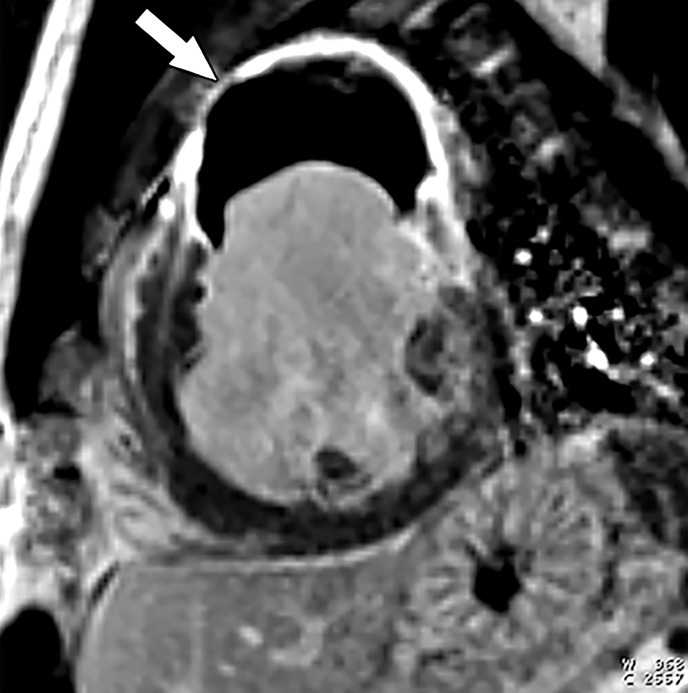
True aneurysm in a 42-year-old man with cardiomyopathy of unknown cause who was referred for further evaluation. (a) Short-axis image from SSFP cine MRI shows a wide-mouthed outpouching (arrow), compatible with an aneurysm, arising from the anterior wall of the LV and containing intermediate-signal-intensity material (*) within it. (b) Short-axis phase-sensitive segmented inversion-recovery LGE image shows diffuse enhancement of the aneurysm wall (arrow), compatible with infarcted myocardium, as well as a low-signal-intensity thrombus within the aneurysm.
